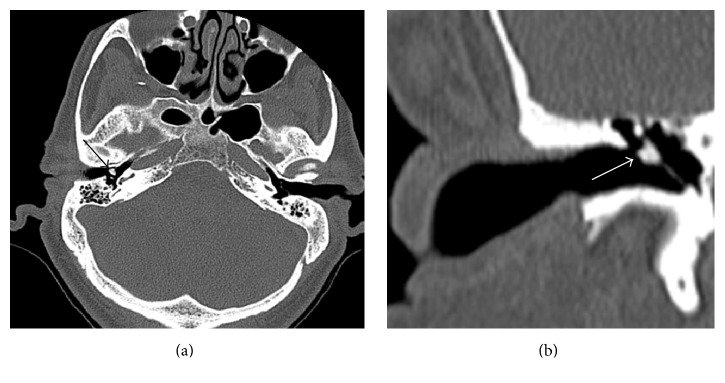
(a) Axial bone windowed temporal bone CT scan showing a 3-4 mm hyperdense lesion (arrow); the middle ear is aerated. (b) Coronal bone windowed temporal bone CT scan showing a 3-4 mm lesion lateral to the neck of the malleus (arrow). The scutum (lateral wall of epitympanum) is free of bone erosion (images courtesy of Banner University Medical Center).
